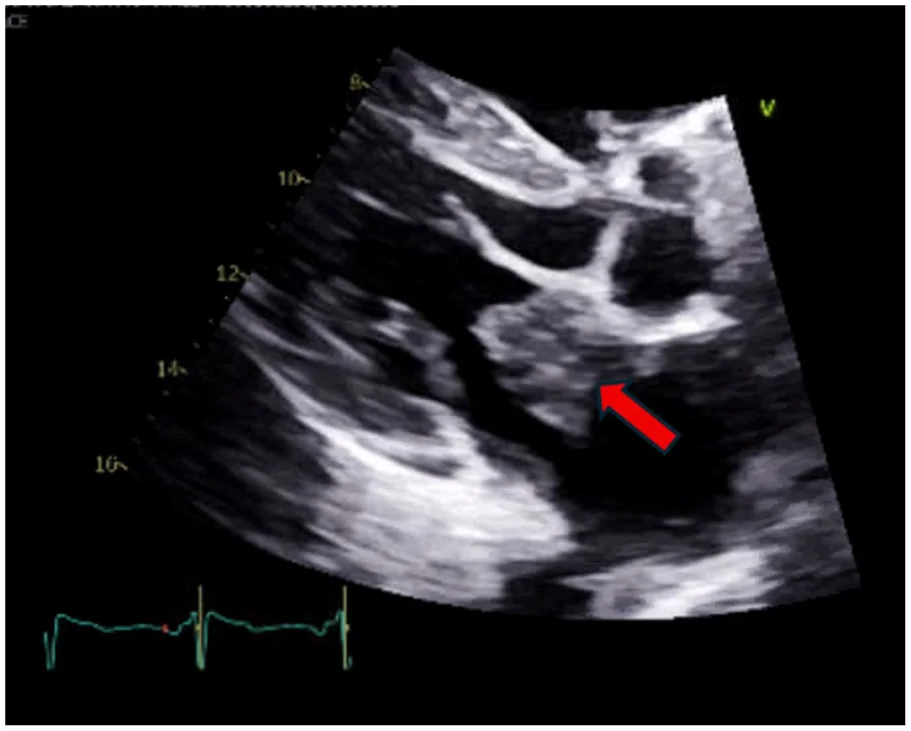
Preoperative transthoracic echocardiogram. Echo images showing a definite medium-size (2.7 cm × 2.7 cm), broad based, spherical, mobile tumor in the atrial cavity (red arrow), attached to the inter-atrial septum very near to the base of the anterior mitral leaflet.
